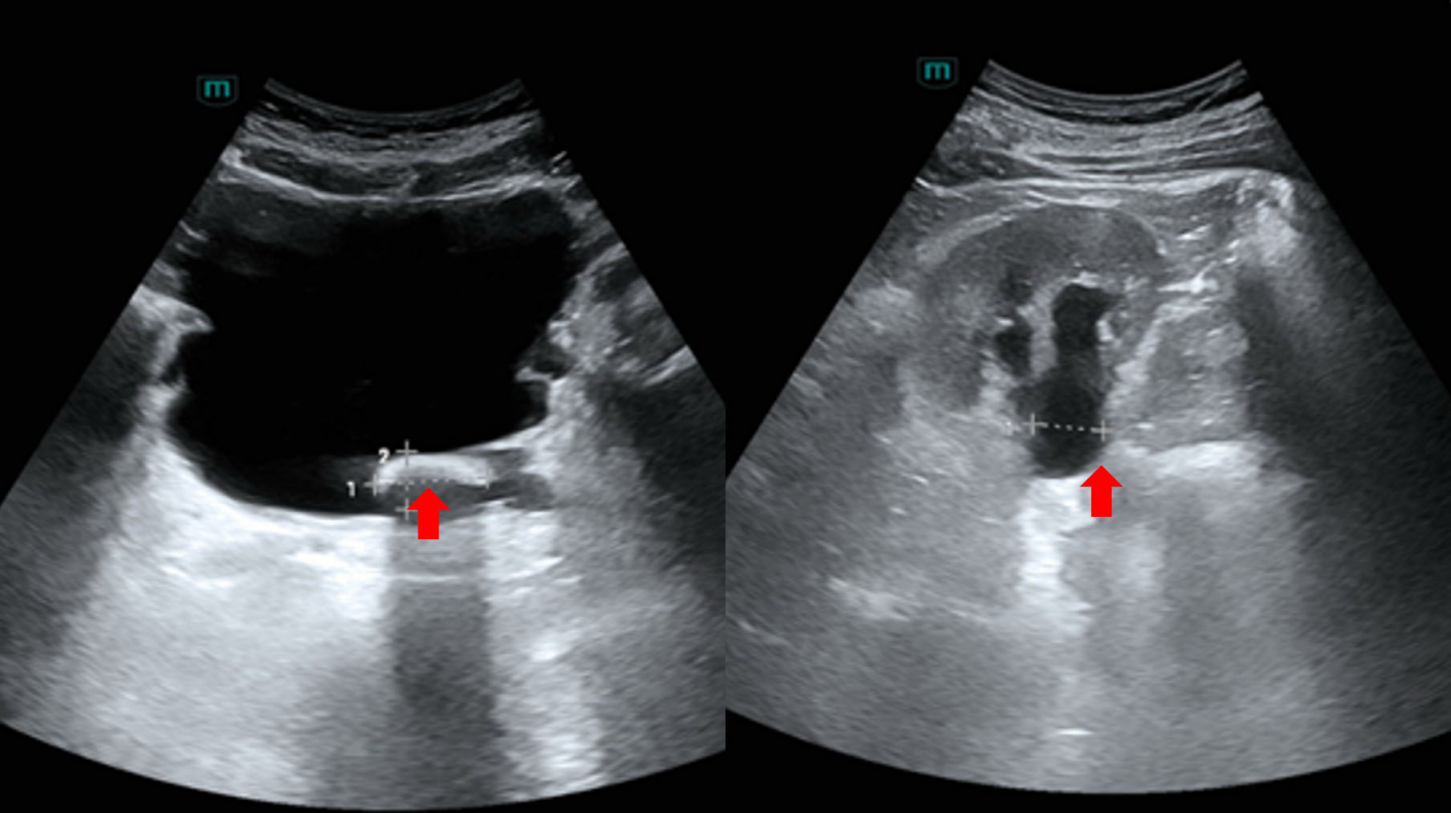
Urological ultrasonography. Both kidneys demonstrated normal size and morphology with clear capsules and homogeneous parenchymal echogenicity. The intrarenal architecture was clearly visualized. Bilateral pelvic separation was observed, measuring approximately 1.7 cm on the right and 1.8 cm on the left, without obvious space-occupying lesions. Bilateral ureteral dilation was noted, measuring approximately 0.9 cm on the right and 0.5 cm on the left. The bladder was well-distended with thickened, irregular walls showing trabeculated changes consistent with detrusor hypertrophy. An intraluminal hyperechoic mass measuring approximately 2.8 cm × 1.4 cm was identified, with posterior acoustic shadowing. The prostate was enlarged, measuring approximately 4.9 cm × 5.6 cm × 4.3 cm, with heterogeneous internal echogenicity and hyperechoic foci at the interface between the inner and outer glands.
